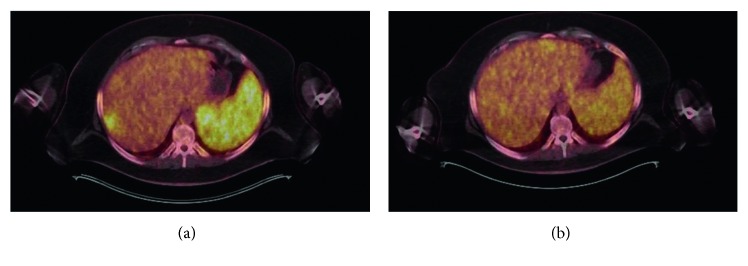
Fused PET-CT images: abdominal window showing resolution of liver and spleen lesions (a) prior to lenalidomide use and (b) after lenalidomide use.
